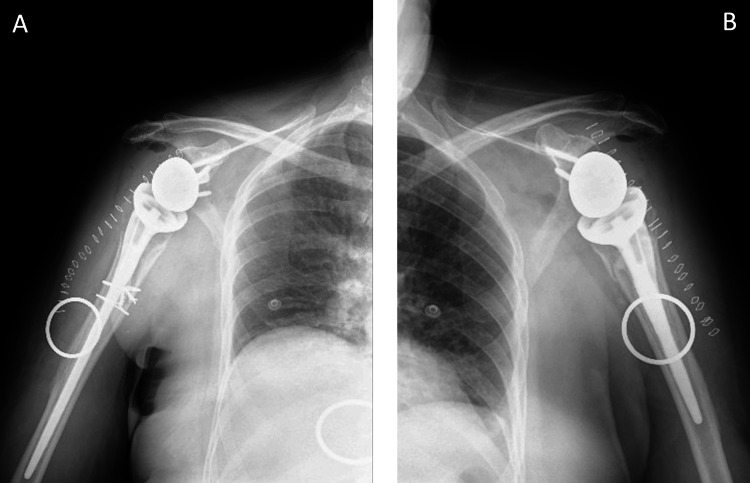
X-ray of the right (A) and left (B) shoulders and humeri (post-operative day 0). (A) Right humerus plain X-ray post-operative anteroposterior view demonstrating reverse total shoulder arthroplasty. (B) Left humerus plain X-ray post-operative anteroposterior view demonstrating reverse total shoulder arthroplasty.
